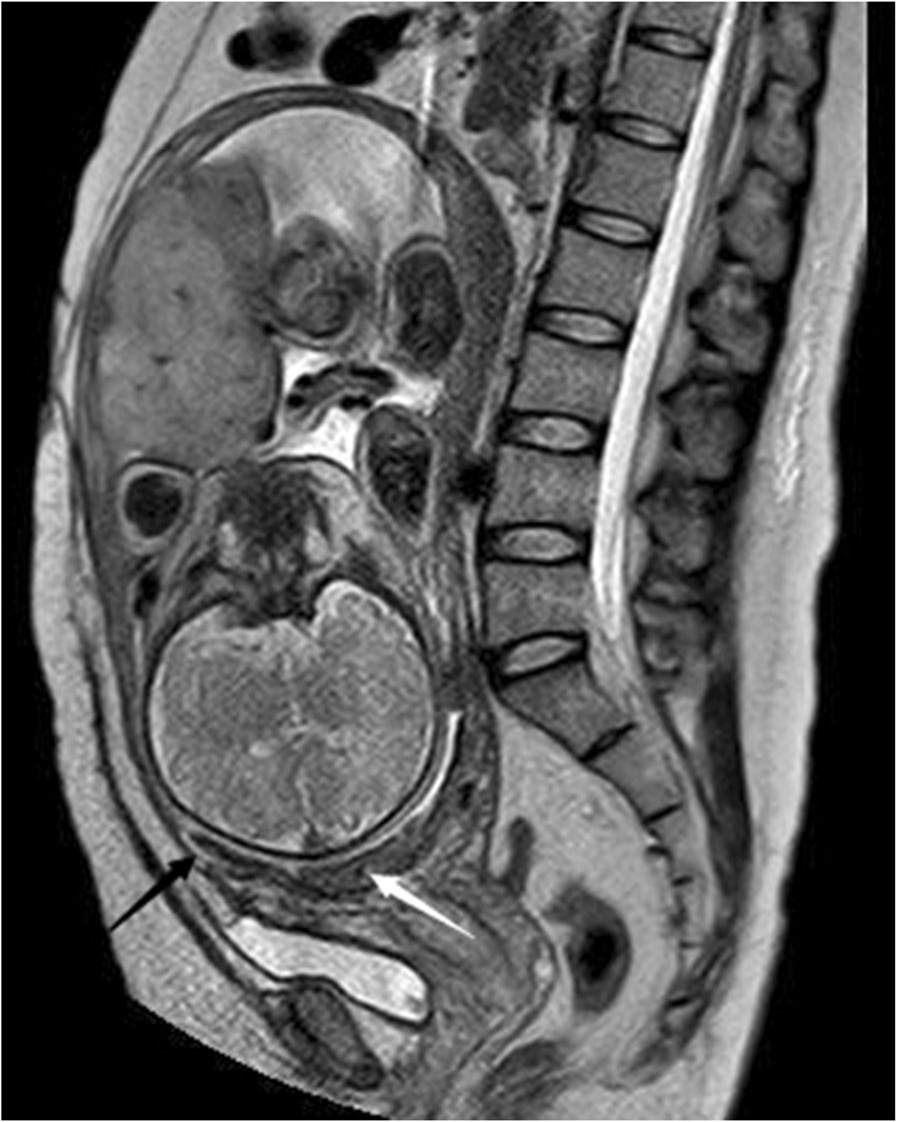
Magnetic resonance imaging findings at 37 weeks’ gestation. Heterotopic complete placenta previa and placenta accrete, dead fetus and placenta covering the internal cervical os (*white arrow*). Thin myometrial layer of cesarean scar (1.3 mm, *black arrow*)
